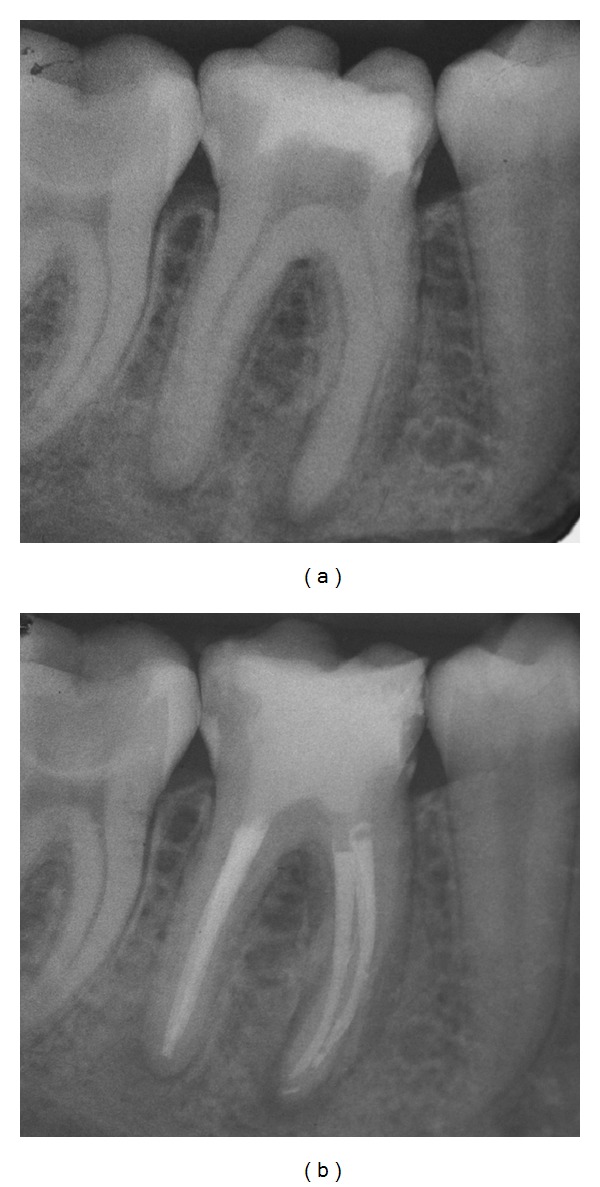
(a) Initial radiograph showing constricted canals mainly in the apical and medium thirds of the mesial root. (b) Final radiograph.
